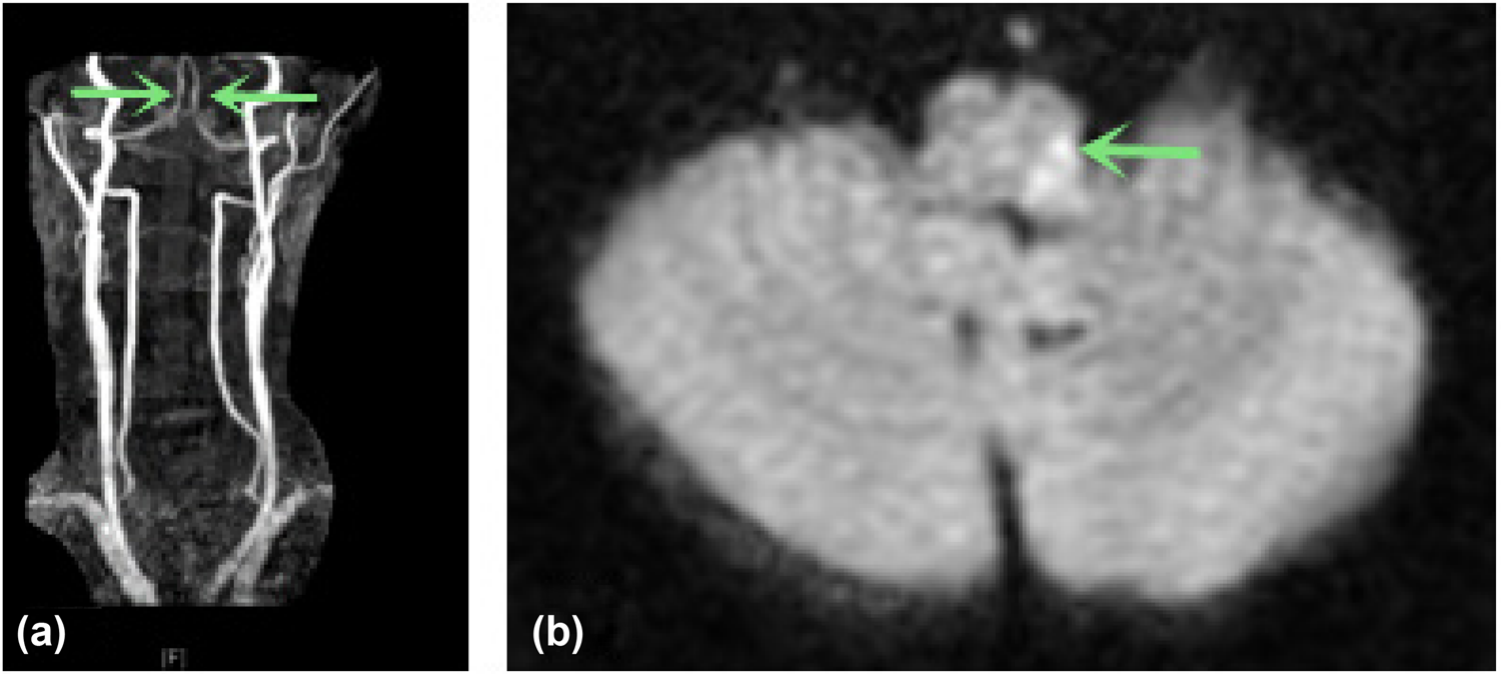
(a) Patent bilateral vertebral arteries (arrow) on CTA neck from three years prior to presentation; (b) admission head MRI (143 min after symptom onset) which showed small punctate infarction in the left medulla oblongata (arrow).
